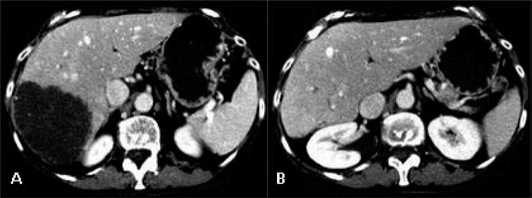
(a) pre-surgical CT shows a huge liver lesion; (b) CT after resection performed after embolization of right portal branch, confirms liver left lobe hypertrophy
